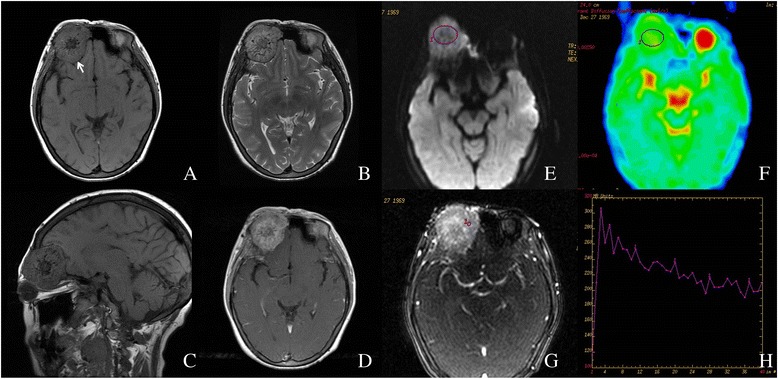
Right orbital paraganglioma in a 36-year-old female. a, b Non-enhanced axial T1W and T2W MR images. c Non-enhanced saggital T2W MR image. d Contrast enhanced axial T1W image. e, f ROI on DWI (*b* value = 1000 s/mm^2^) and corresponding ADC map. The average ADC value of this lesion was 1.196 × 10^−3^ mm^2^/s. g, h DCE-MRI and corresponding TIC, which showed a washout pattern
